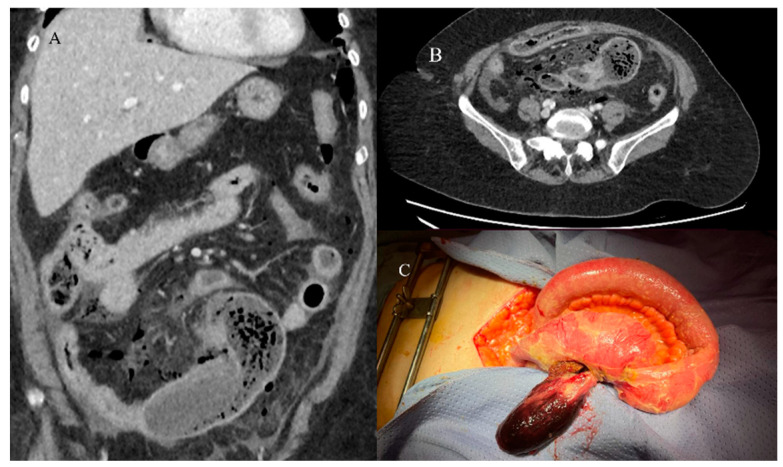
CT images from the previous case of the 72-year-old woman with an intestinal polyp and SBO show signs of necrosis and perforation. Initially, nonsurgical management was decided upon; however, the intestinal polyp became complicated with necrosis and perforation. Signs of perforation with free air are visible on the coronal plane in image (A) and on the axial plane in image (B). During surgery in image (C), a necrotic ileal polyp with intestinal laceration was found.
